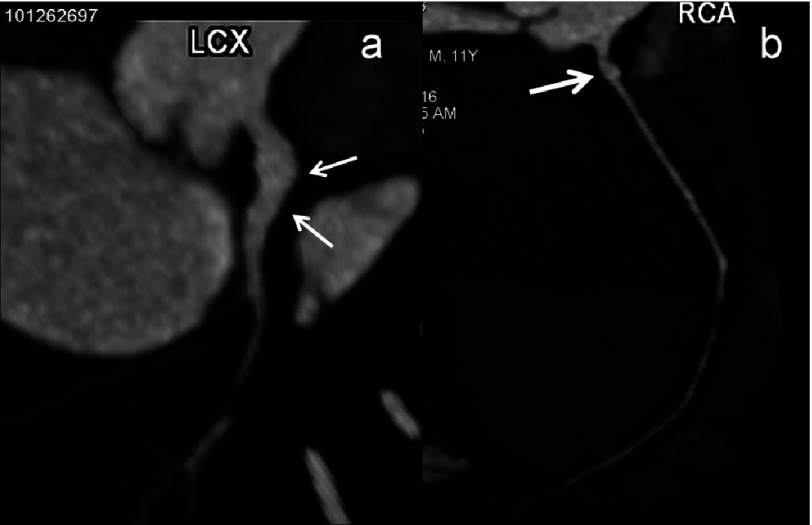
(a–b): CTCA curved maximum intensity projection (MIP) images of a 11 year male child in convalescent phase (5 years after diagnosis) show fusiform aneurysmal dilation (a - thin arrows) of proximal left circumflex artery (LCX) and saccular aneurysm of proximal RCA (thick arrow). Left circumflex artery is usually not well evaluated on 2D- transthoracic echocardiography.
